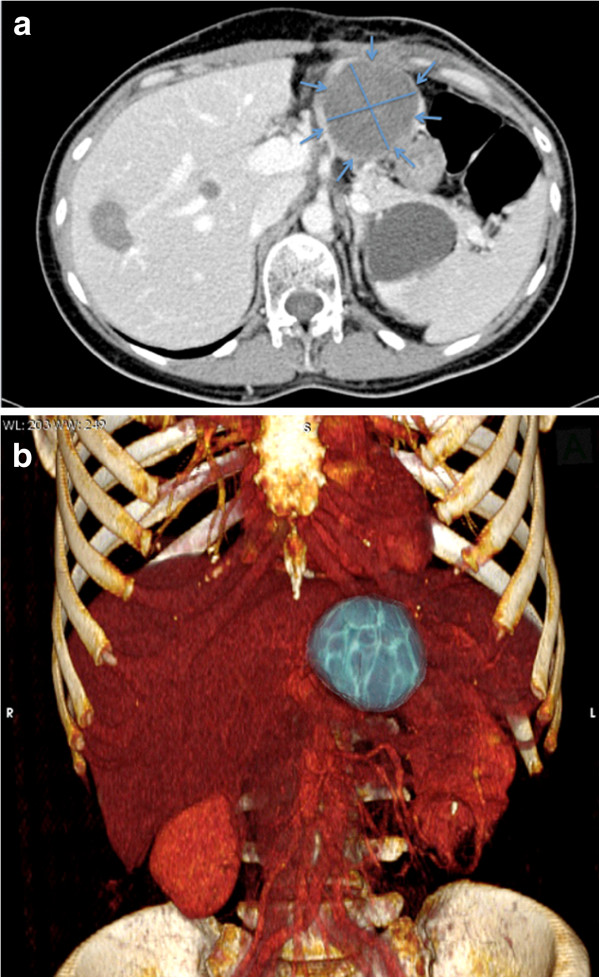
CT of patient 3 months after treatment. (a). Contrast-enhanced multi-detector CT. A 6 cm × 6 cm liver lesion (blue arrows) is identified, showing a decrease in size of 72%. (b) 3D rendering image post-treatment.
